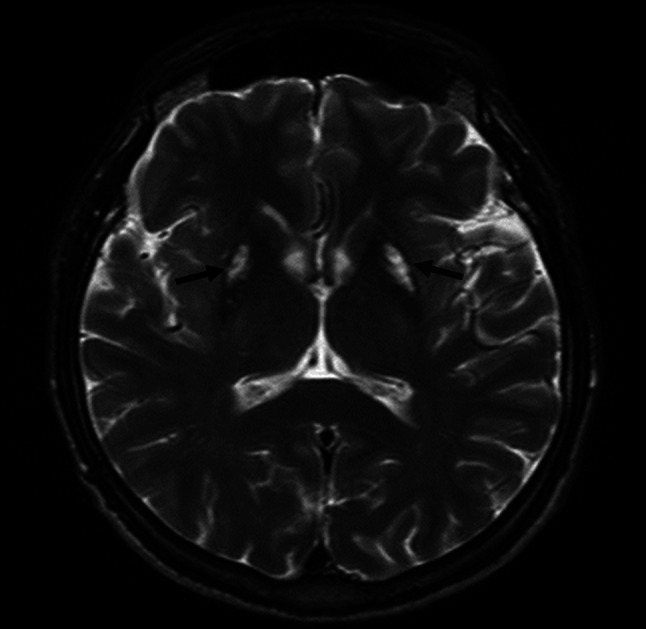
The MR image mimicking the “eye of the tiger” sign in T2-weighted magnetic resonance sequences; bilateral hypointensity in both putaminal regions and lateral parts of globi pallidi, with a central hyperintensity (*black arrows*)
